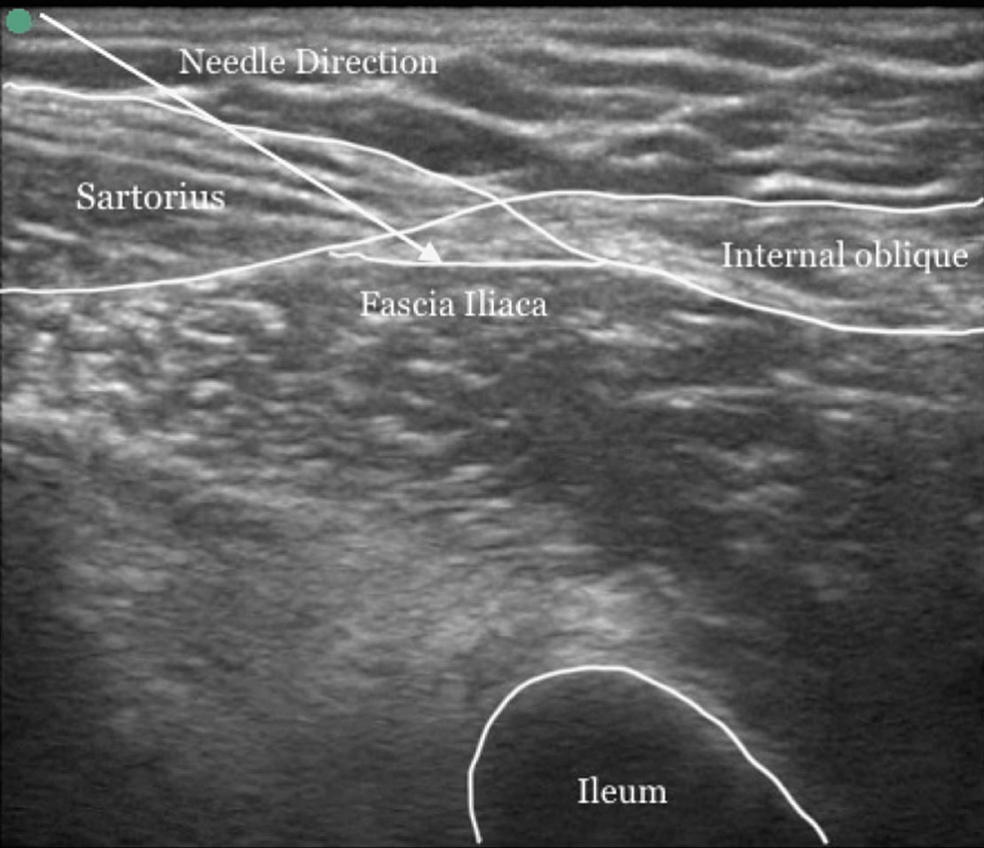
Ultrasound image of SIFICB. The appearance of the "BOW-TIE" sign formed by the sartorius and internal oblique muscles. The white arrow shows the direction of the needle to pierce the fascia iliaca. SIFICB: suprainguinal fascia iliaca compartment block
